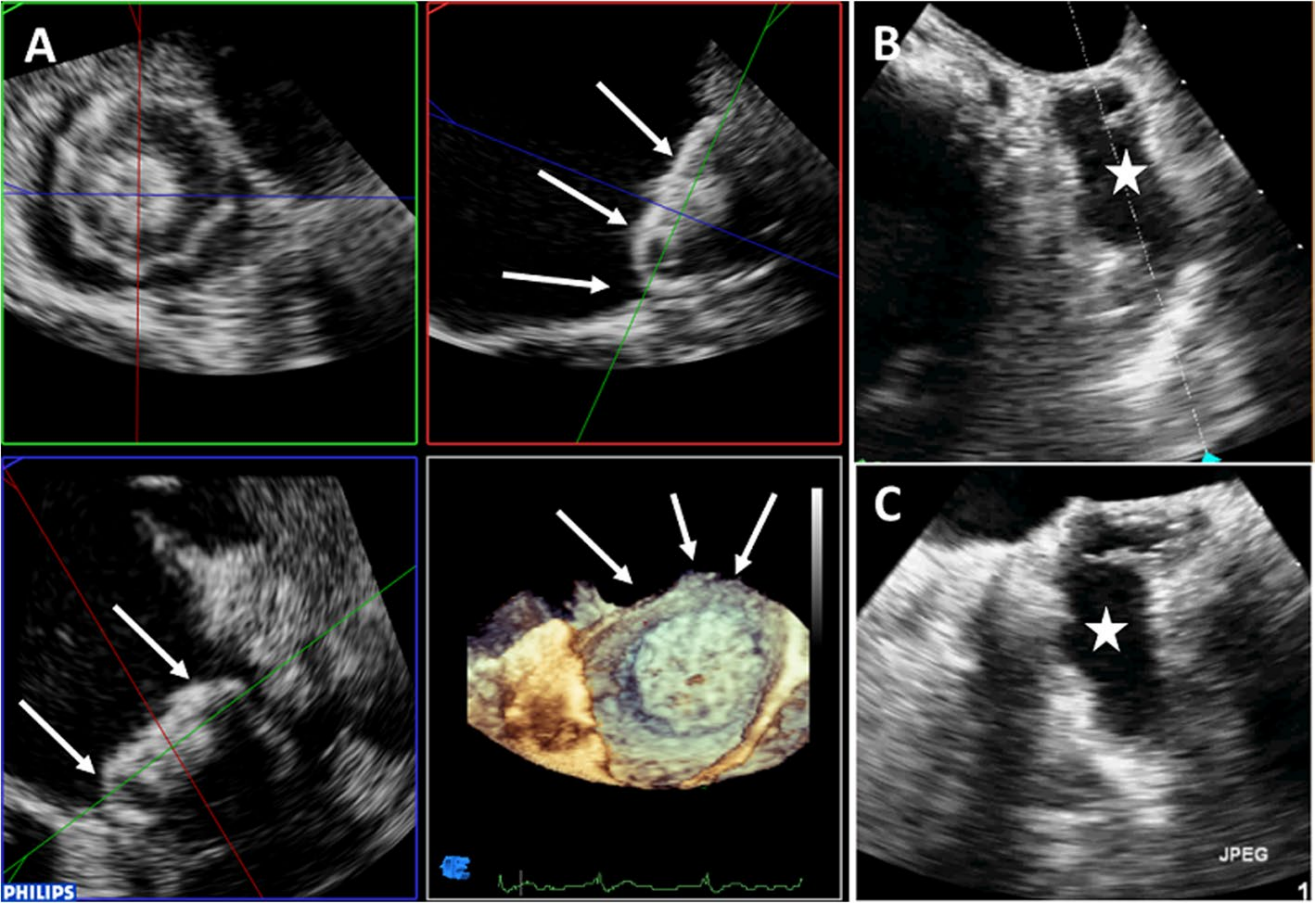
F (A) Quad-screen acquisition using three-dimensional transthoracic echocardiography shows a Watchman (Boston Scientific) closure device of the left atrial appendage (white arrows). B, C Left atrial appendage closed surgically (star)
